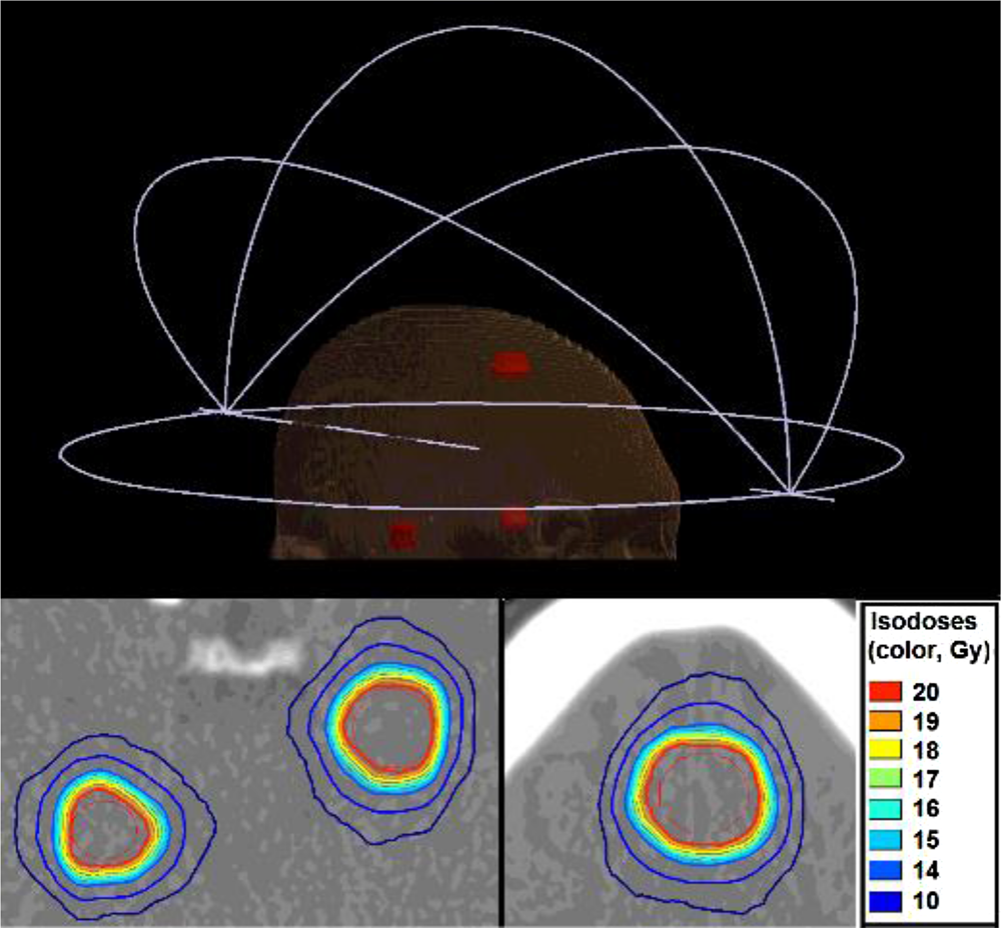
An indicative reference treatment plan (i.e., no leaf offset applied) involving three targets (metastases). Arcs configuration is presented (top) and isodose lines corresponding to reference dose distributions, are superimposed on axial slices (bottom) of the planning CT scan
